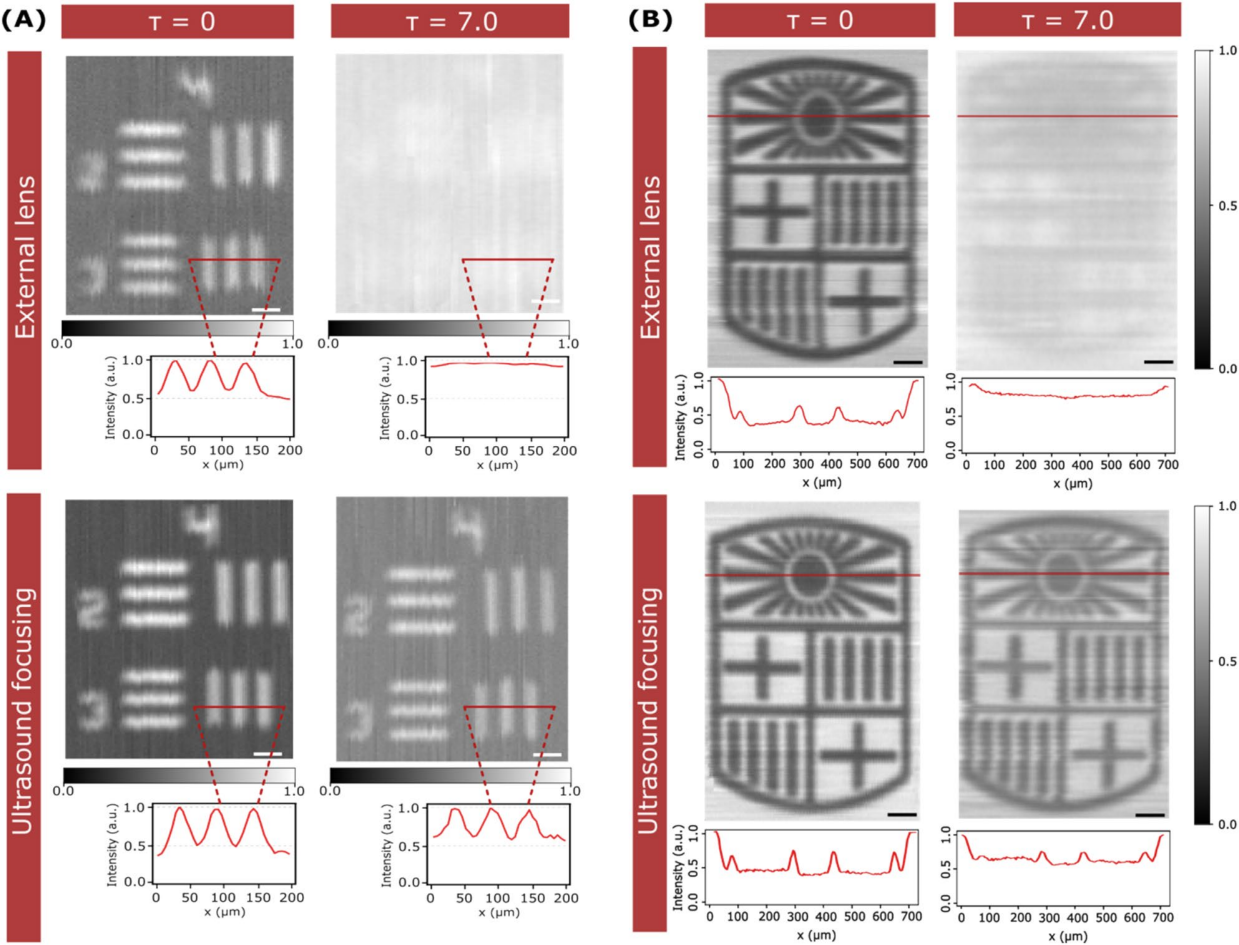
Imaging with ultrasound-focused light. (A) Reconstructed images of a USAF target group 4 elements 2 and 3 acquired by scanning the sample, point-by-point, across the focus obtained with an external lens (top) and ultrasound focusing (bottom) inside a homogeneous medium (left) and a turbid medium with τ = 7.0 (right). The insets correspond to the intensity profiles of the selected regions. Scale bars are 50 μm. (B) Reconstructed images of the UB (Universitat de Barcelona) logo acquired under the same conditions as (A). The insets correspond to the intensity profile highlighted with a line. All images are obtained in reflection mode. Scale bars are 100 μm.
